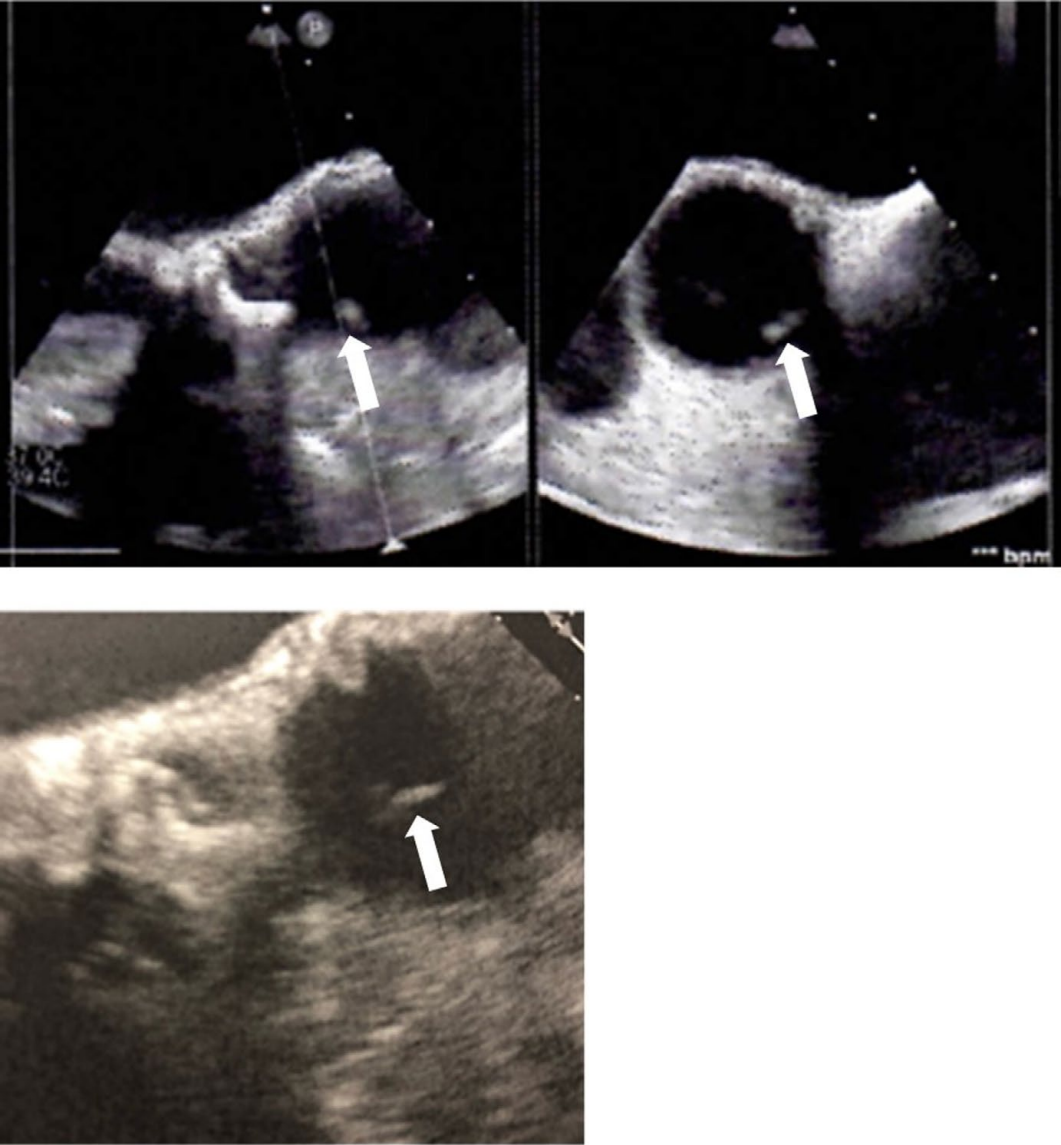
Trans‐esophageal echocardiogram showing a vegetation attached to the aortic valve (white arrows, left: mid‐esophageal long‐axis view; right: short‐axis view; under: mid‐esophageal long‐axis view magnified image)
